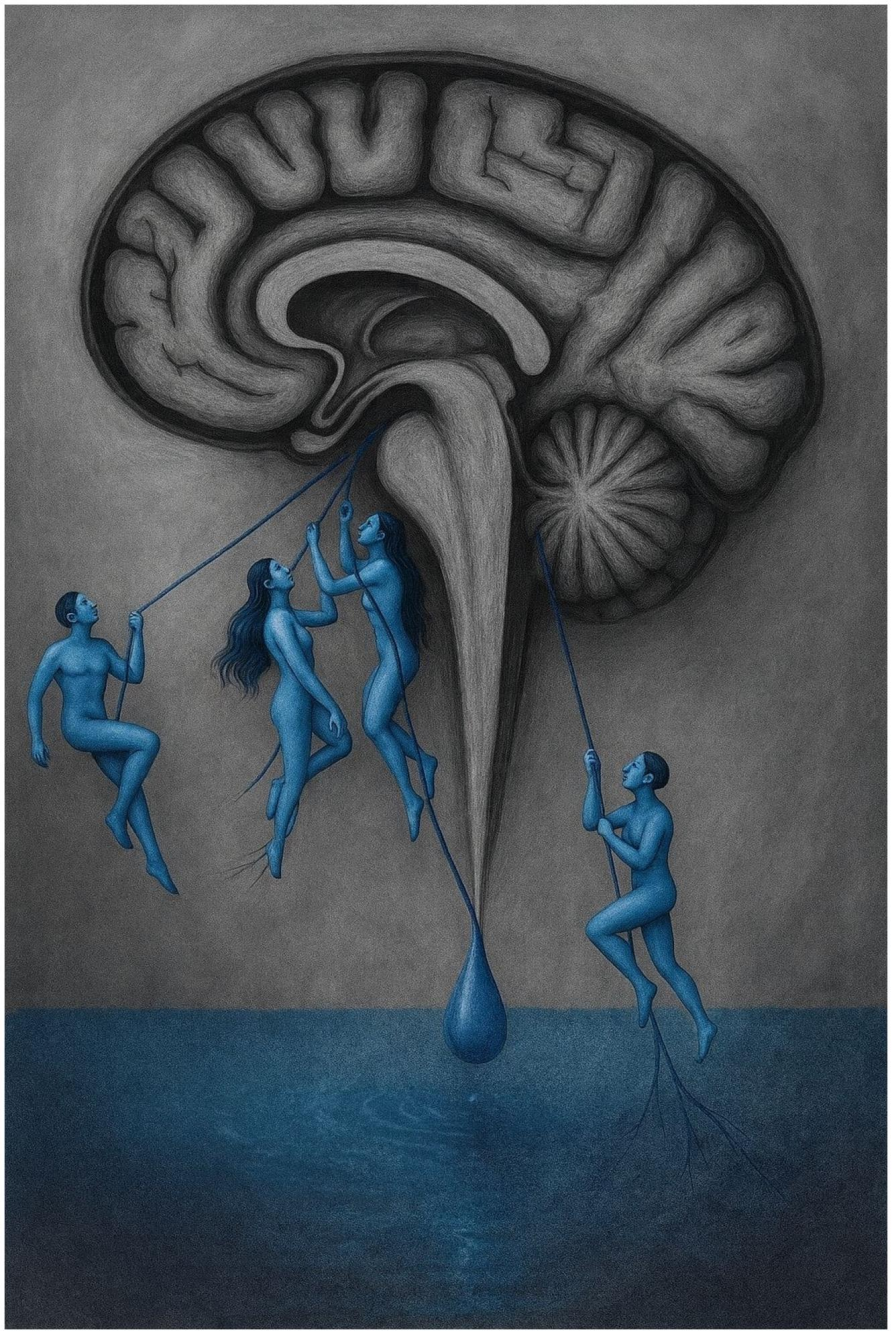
Human-like forms of leaking cerebrospinal fluid pull the brain downward, reflecting brain sagging and tonsillar herniation on MRI.
